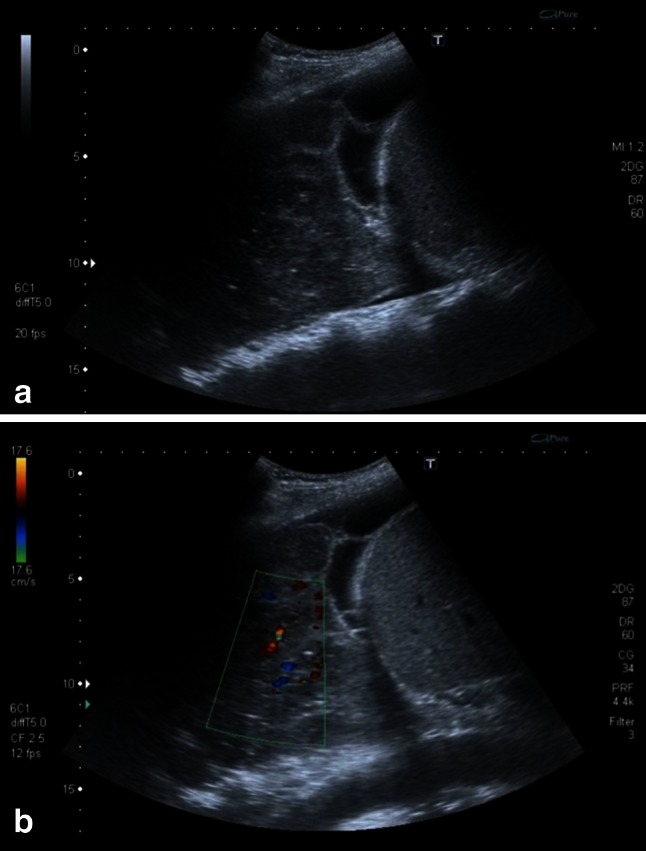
a Sonographic image of a right-sided consolidation. The consolidation shows areas of lower echogenicity suggesting lymphoma infiltration. Additionally a pleural effusion with fibrin strands is present. b Using color-Doppler the hypoechoic areas surround the vasculature (see Online Resources 6 and 7)
